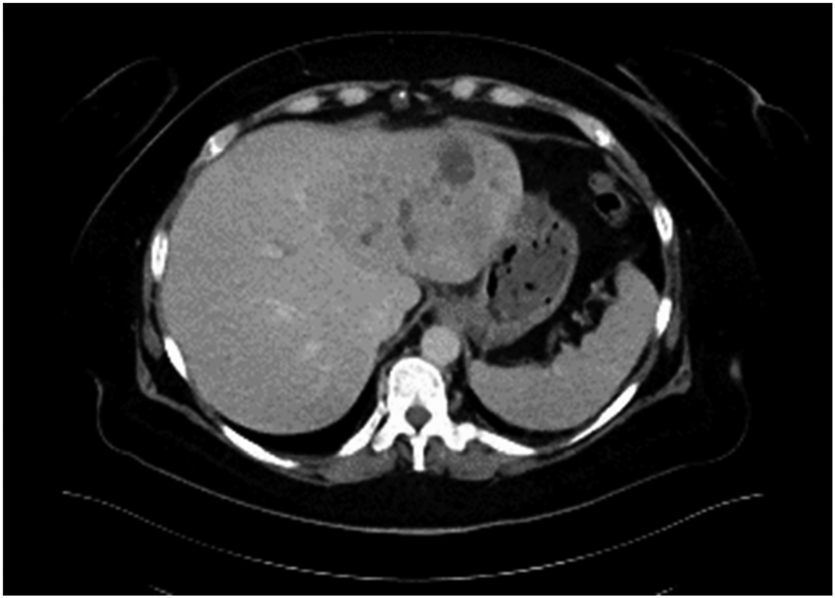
CT scan after antibiotic treatment and bile duct stenting preoperatively.
